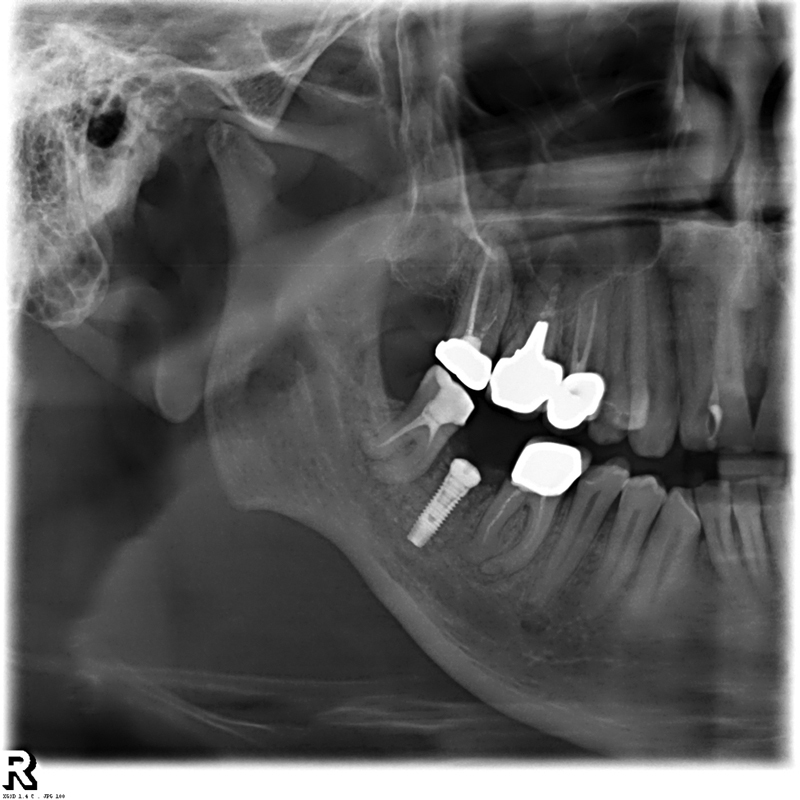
Half-panoramic X-ray showing the correct endosseous implant position and perfect integration of the bone graft.
